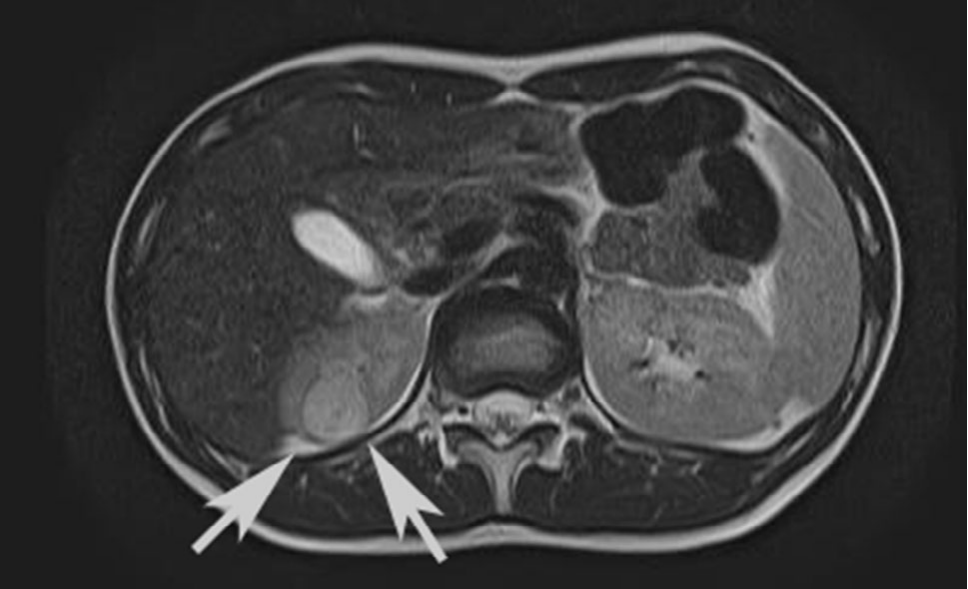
MRI showing a hyperintense lesion on the T2‐weighted image. Arrows: tumor.
